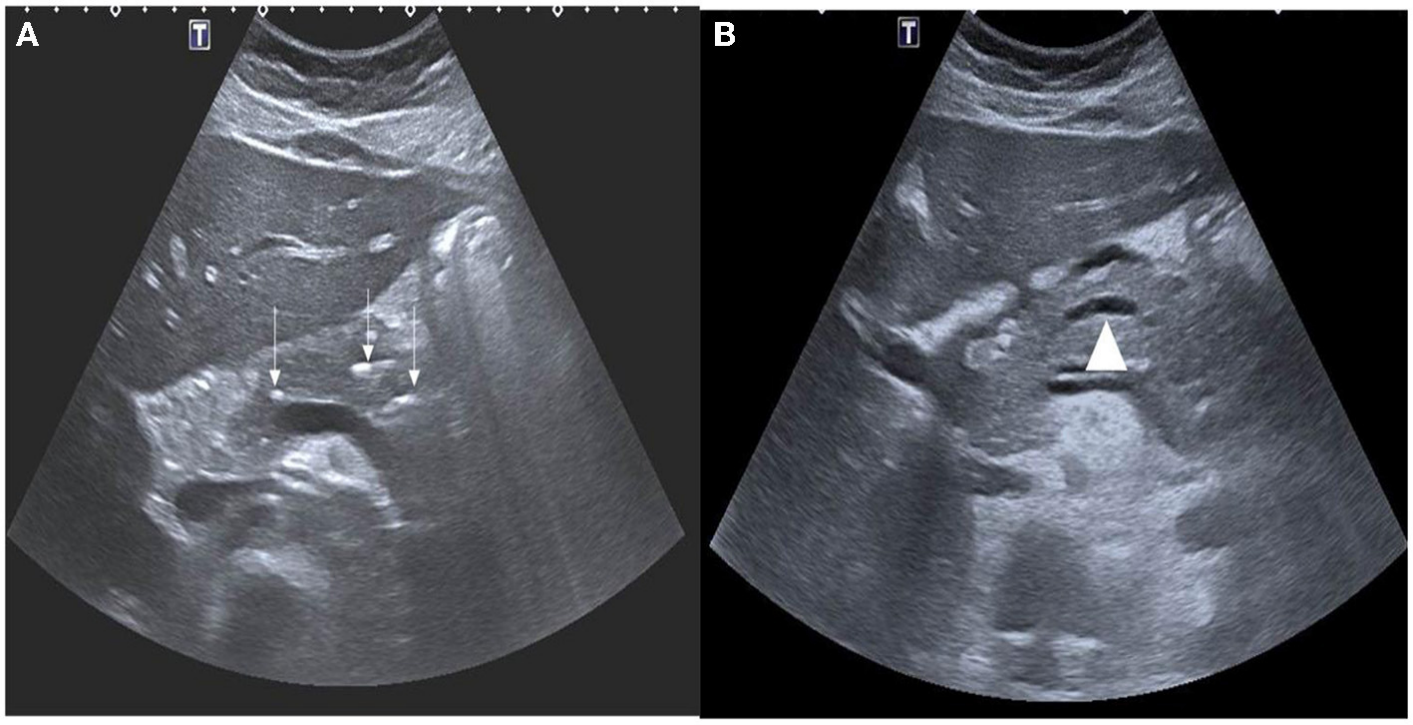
(A) Axial ultrasound images demonstrating multiple foci of calcifications in the pancreas (white arrows). (B) Notice also the dilated main pancreatic duct (arrow head).
